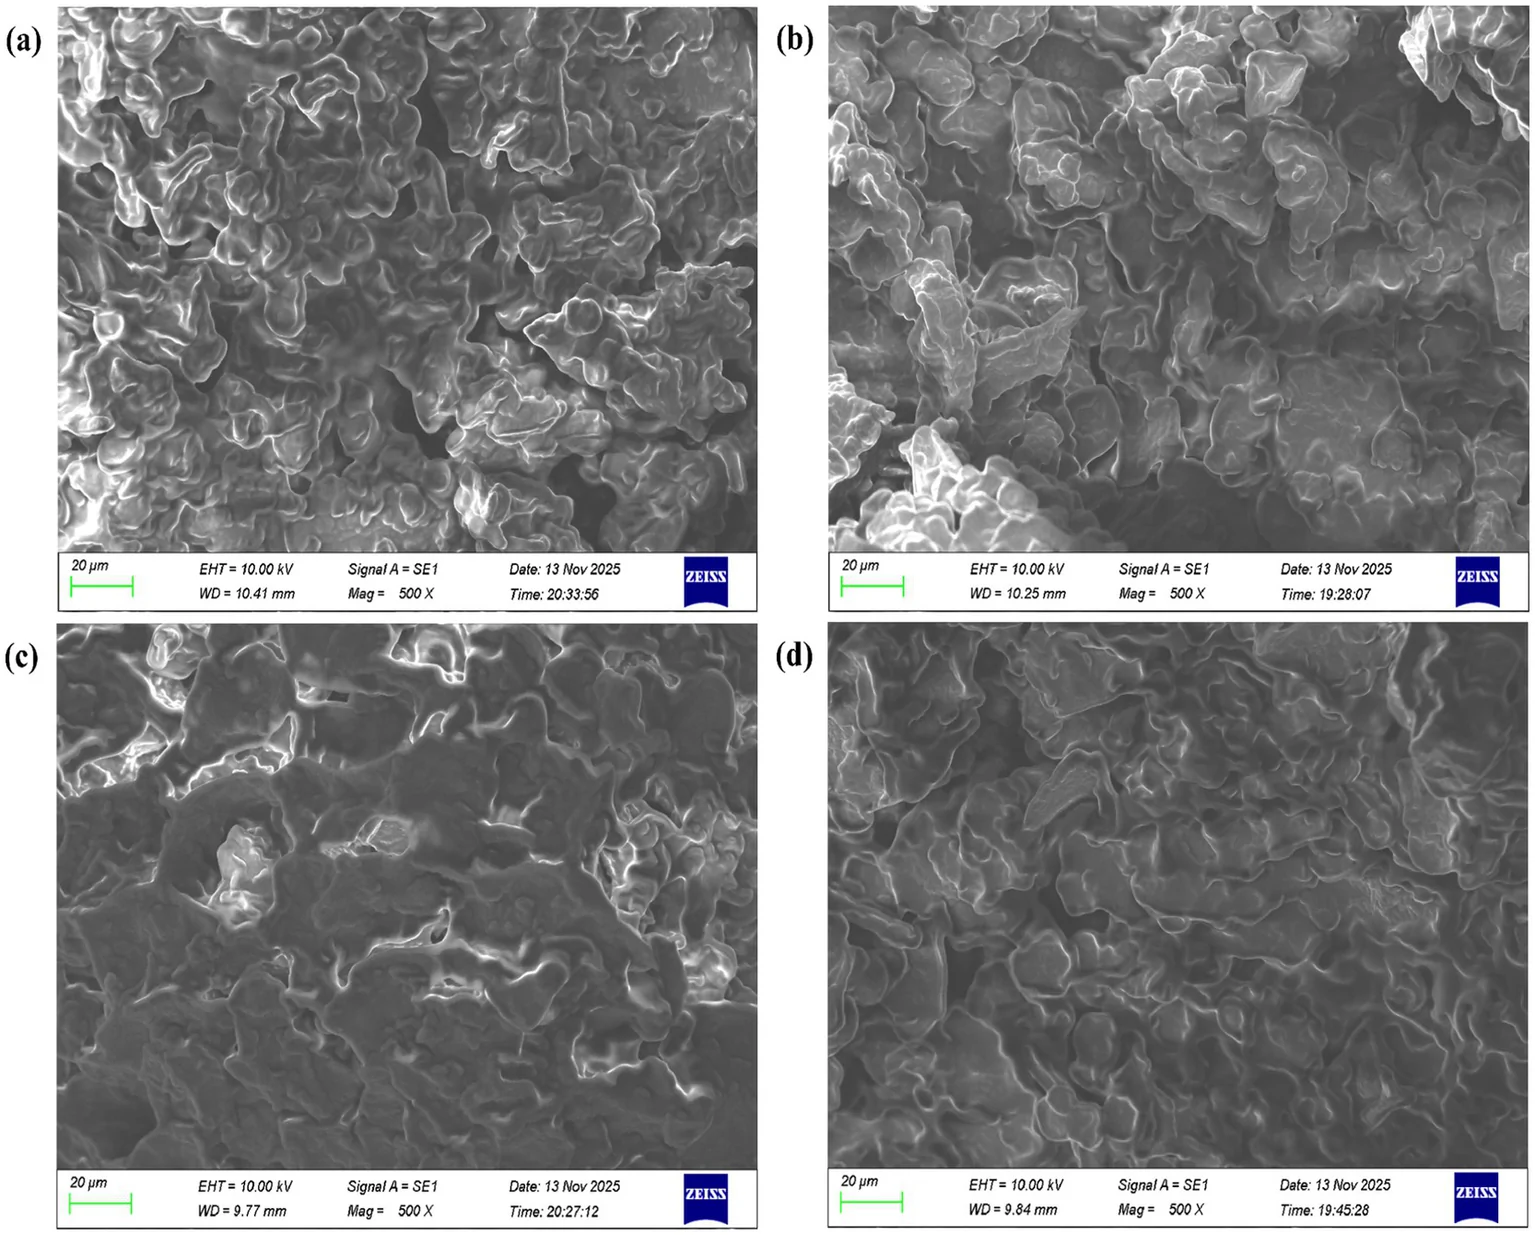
SEM analysis images of *Akebia trifoliata* seeds under different pretreatments: (a) Unpretreated (Ud), (b) Without ultrasonic pretreatment with ethanol (60%) (Et), (c) Ultrasonic treatment (200 W, 30 min without ethanol pretreatment) (UL), (d) Ultrasonic ethanol pretreatment (200 W, 30 min, 60% ethanol) (UL-Et).
